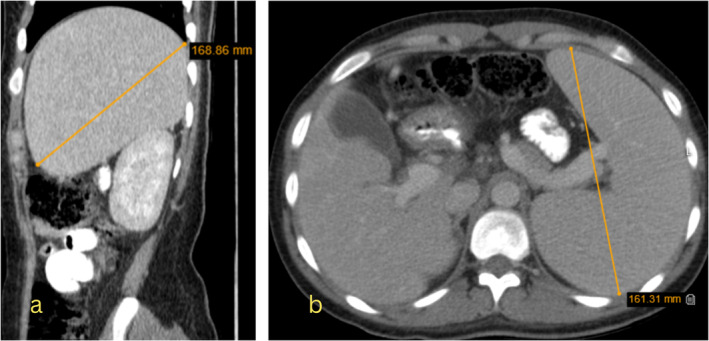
Non‐contrast CT scan with mildly heterogenous liver, which appears normal on the post‐contrast image with enlargement of the spleen. (a) sagittal view, (b) axial view.
